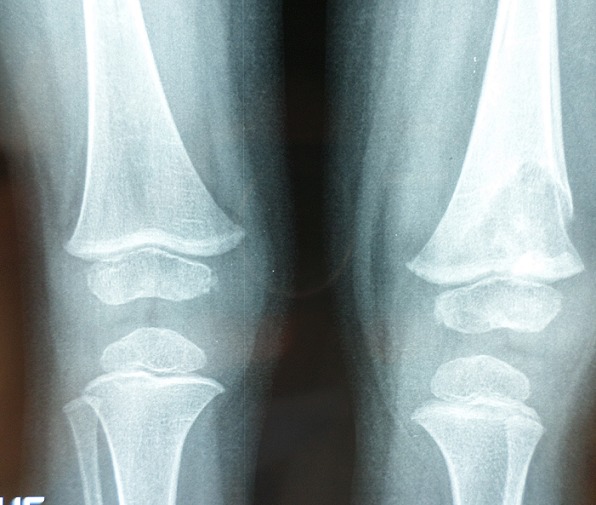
Radiographie standard du fémur gauche montrant une lésion ostéolytique
